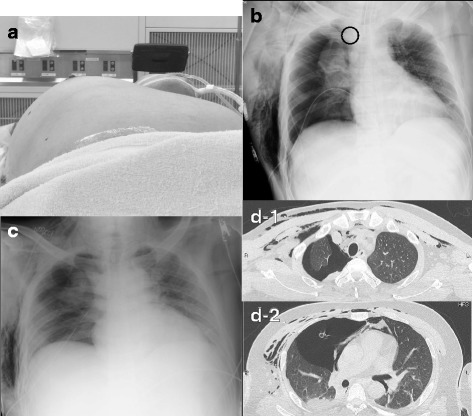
Postoperative images. a Significant chest distention on the right side. The photograph was taken after needle thoracentesis. b Chest X-ray showing right pneumothorax, heart shifted to the left, massive subcutaneous emphysema, and malpositioned chest drainage tube, which had to be re-positioned. c Correct placement of the drainage tube. d-1, d-2 Chest CT showing subcutaneous and mediastinal emphysema, right pneumothorax, and no bullae or blebs
